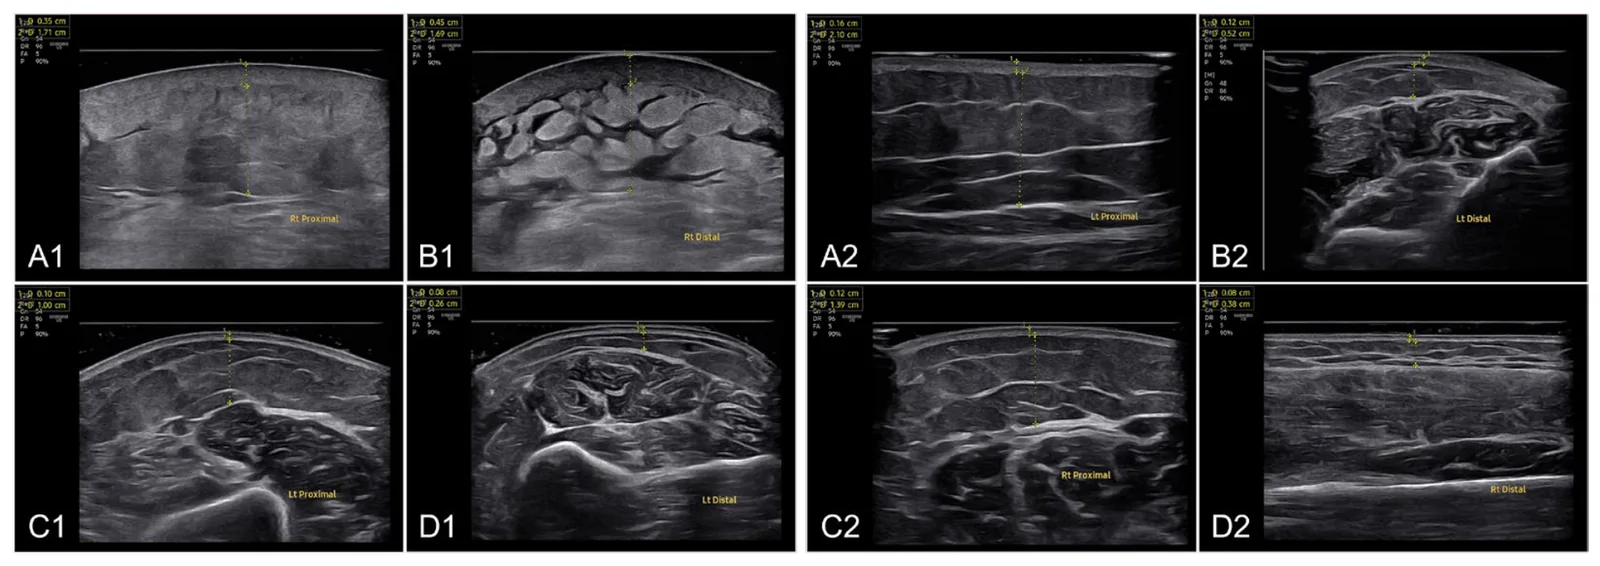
Representative ultrasound images of dermal and subcutaneous thickness measurements of patients with clinical and subclinical lymphedema. (A1–D1) Images of a representative patient with clinical lymphedema: ipsilateral upper arm (A1), ipsilateral forearm (B1), contralateral upper arm (C1), and contralateral forearm (D1). (A2–D2) Images of a representative patient with subclinical lymphedema: ipsilateral upper arm (A2), ipsilateral forearm (B2), contralateral upper arm (C2), and contralateral forearm (D2). The “+” symbols represent the measurement calipers, and the dotted lines connecting the calipers indicate the measured tissue thickness.
